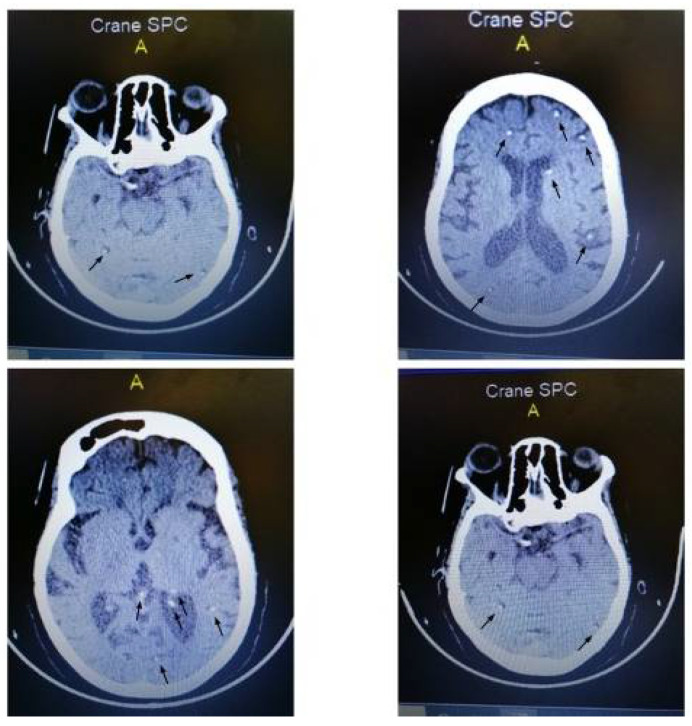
Brain CT scan showing multiple, randomly distributed, round cystic lesions with white dot sign of 4–8 mm in diameter.
